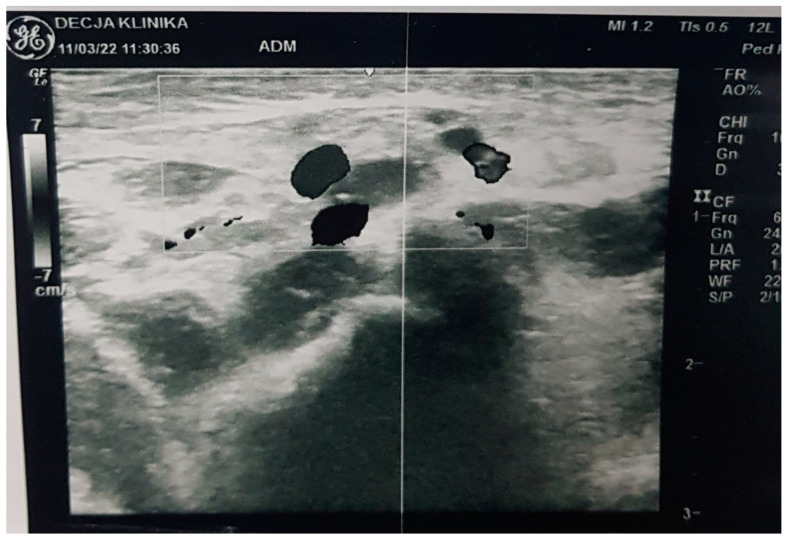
Ultrasound finding of blood vessels: The thrombosis of the femoral vein and iliac vein, on the right side, in newborn 1. The right femoral vein, right side, has a reduced diameter, long with 9 mm, lumen up to 50%, with a pre-obstructive dilatation of the blood vessel of about 5 mm. The right iliac external vein is from the level v. femoral along about 20 mm long filled with heteroechoic contents, more hyperechoic contents, corresponding to the thrombosis. A. femoris and a. iliaca external are preserved flow.
